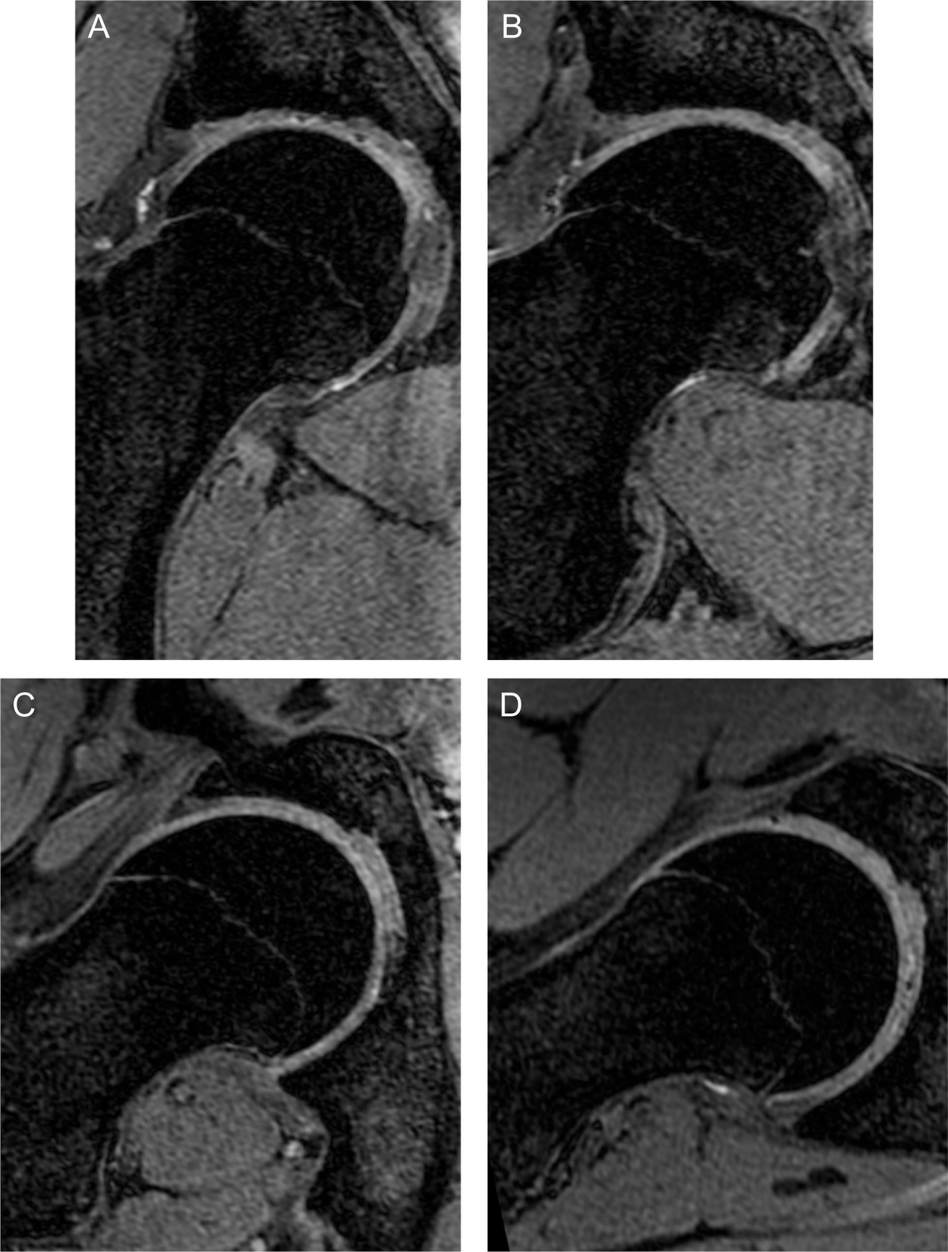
Three-dimensional water selective fluid (WATSf) MRI of SFC player aged 16 years with pronounced cam morphology. Epiphyseal hypertrophy and extension is maximal at 1 o’clock but also present in adjacent positions: 12 o’clock (A), 1 o’clock (B), 2 o’clock (C) and 3 o’clock (D).
